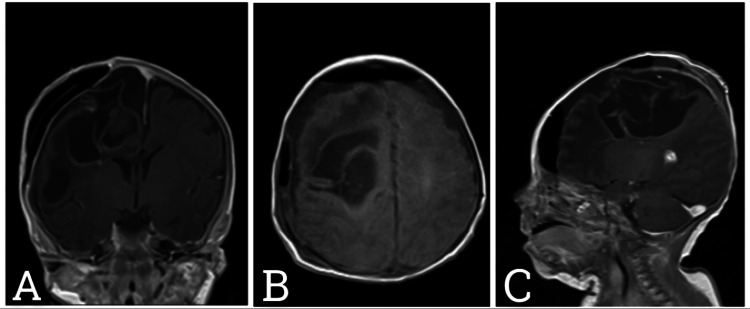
Postoperative T1-weighted MRI brain Coronal (A) Axial (B) Sagittal (C) views shows significant decompression of the large right cerebral cystic mass with almost no mass effects identified in the current MRI examination and no midline shift with normal configuration of the supratentorial and infratentorial ventricular system. Resection of the previously visualized well-defined rounded solid mass with no residual identified in the current MRI examination. Multiple hemorrhagic spots, pneumocephalus and mild subdural fluid are identified related to operative interference.
